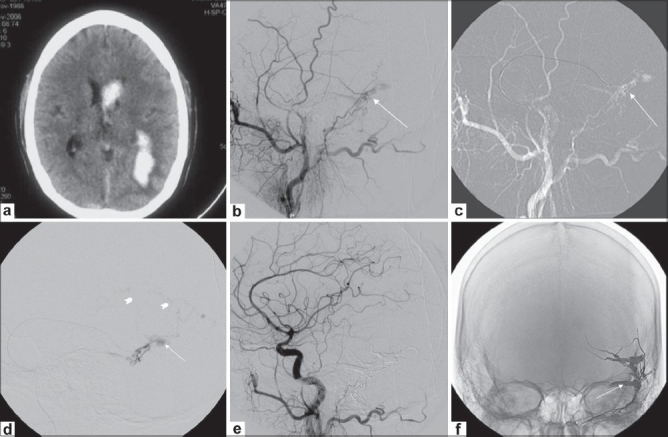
Left petrosal dural arteriovenous fistula (AVF). CT scan of the brain (a) shows left parieto-occipital hemorrhage with intraventricular extension Left external carotid angiogram (b) shows a left petrosal dural AVF (arrow). Left external carotid angiogram (c) with a road map image shows the final position of the microcatheter (arrow) in the middle meningeal artery. Microcatheter angiogram (d) shows the isolated petrosal sinus (arrow) with extensive cortical venous reflux (arrowheads). 2 mL of Onyx 18 was injected from this site over 40 min. Postembolization left common carotid angiogram (e) shows exclusion of the fistula. Plain skull radiograph (f) shows the Onyx cast (arrow)
